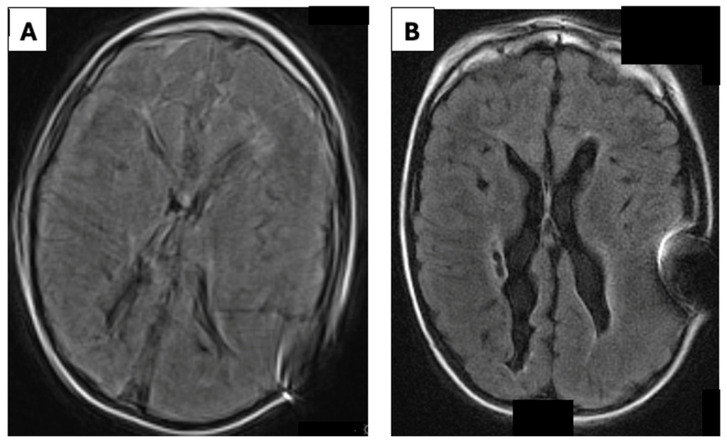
Rapid MRI (A) with axial T2-weighted fluid-attenuated inversion recovery (T2-FLAIR) sequences showing persistent overdrainage in an 8-year-old boy despite multiple valve adjustments. The consequence of this imaging is the surgical replacement of the defective valve. The postoperative Rapid MRI control (B) with T2-FLAIR showing the normalization of the ventricular size.
